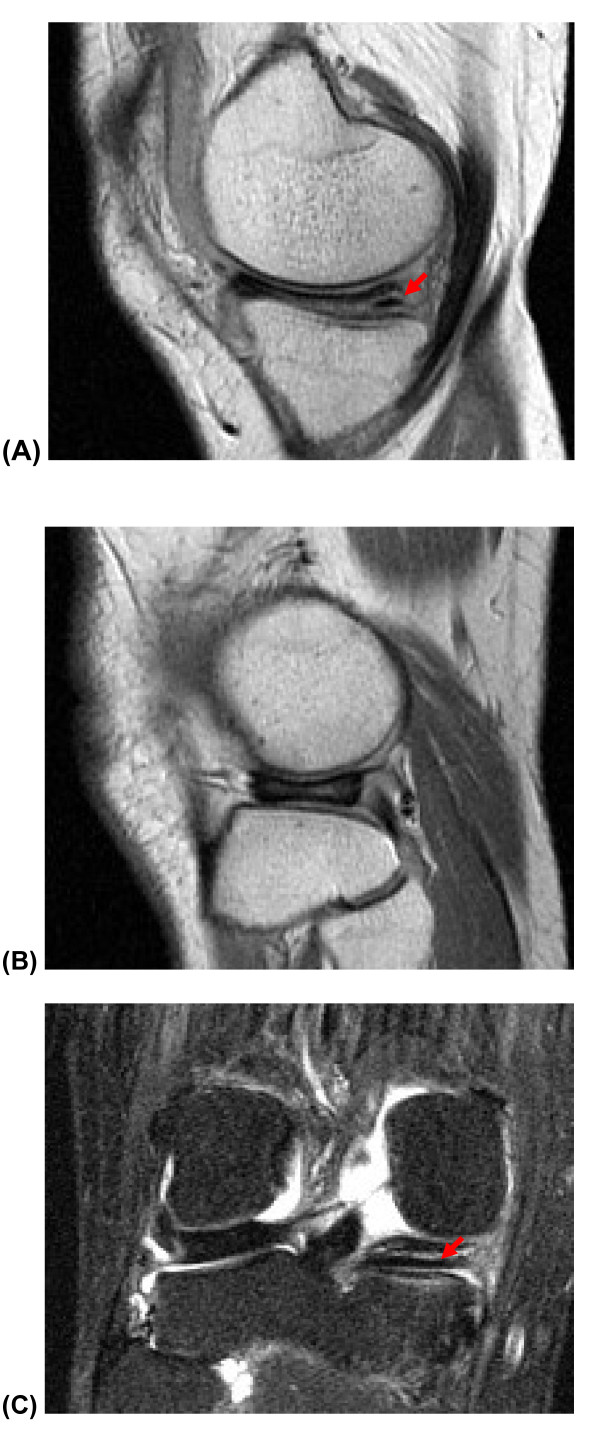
MRI image of the right knee (A) T1-weighted sagittal MRI of the right knee showing medial discoid meniscus with a horizontal tear (indicated by red arrow) and (B) showing lateral discoid meniscus; whereas (C) the T2-weighted coronal MRI showing medial discoid meniscus with tear (indicated by red arrow) and lateral discoid meniscus with no tear.
